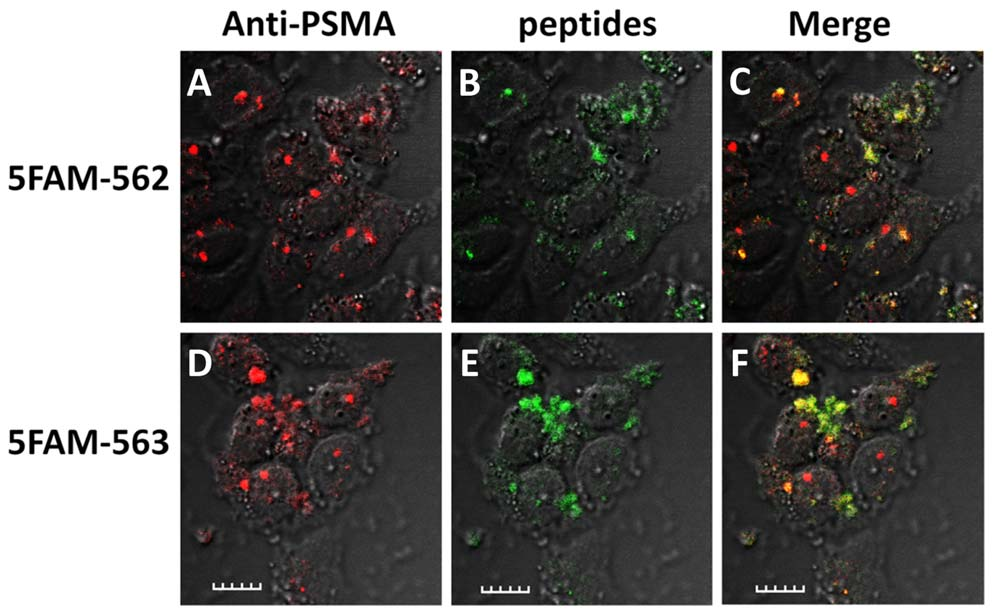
Binding and internalization of 5FAM-562 and 5FAM-563 in LNCaP cells. 5FAM-562 (1 µM, *A–C*, green) and 5FAM-563 (1 µM , *D–F*, green) bound and internalized in LNCaP cells and co-localized with PSMA in intracellular vesicles visualized with anti-PSMA-Mab (red, scale is 20 µm).
